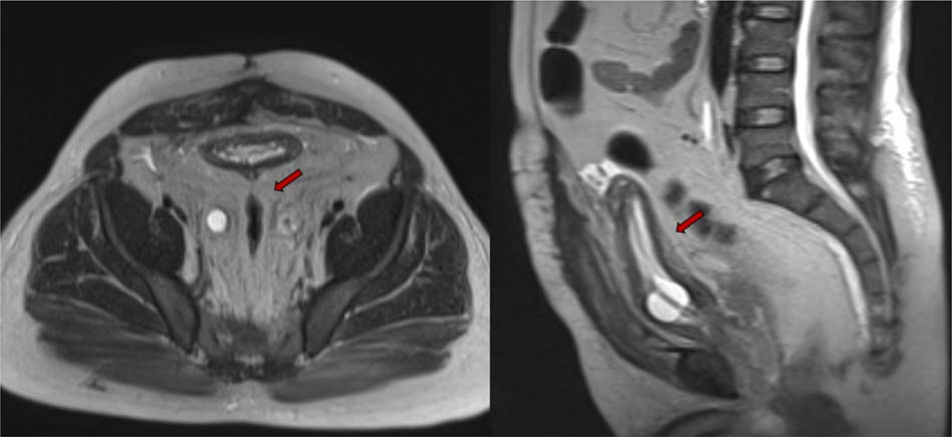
Abdominopelvic MRI T2WI PL surrounding urinary bladder and rectum (red arrow).
